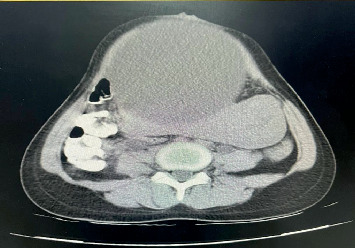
Abdominal computerized tomography (CT) scan showing an enlarged bladder and displaced uterus.
